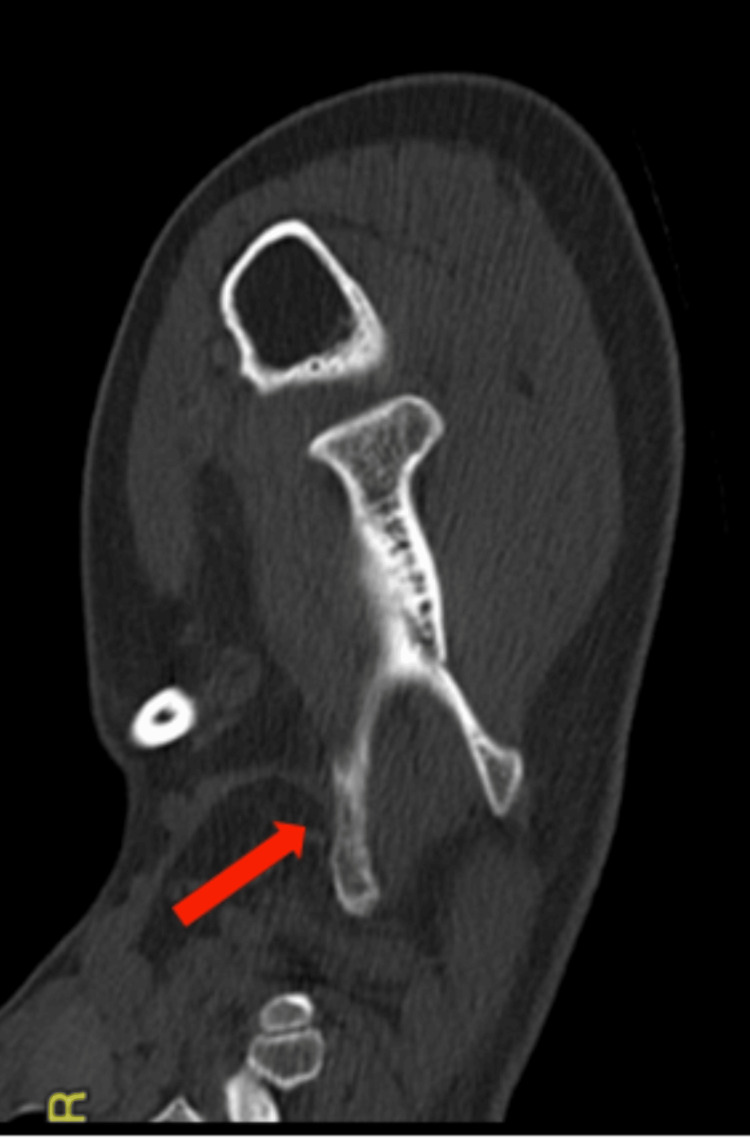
Postoperative CT scan showing the excised tumor mass CT: computed tomography
